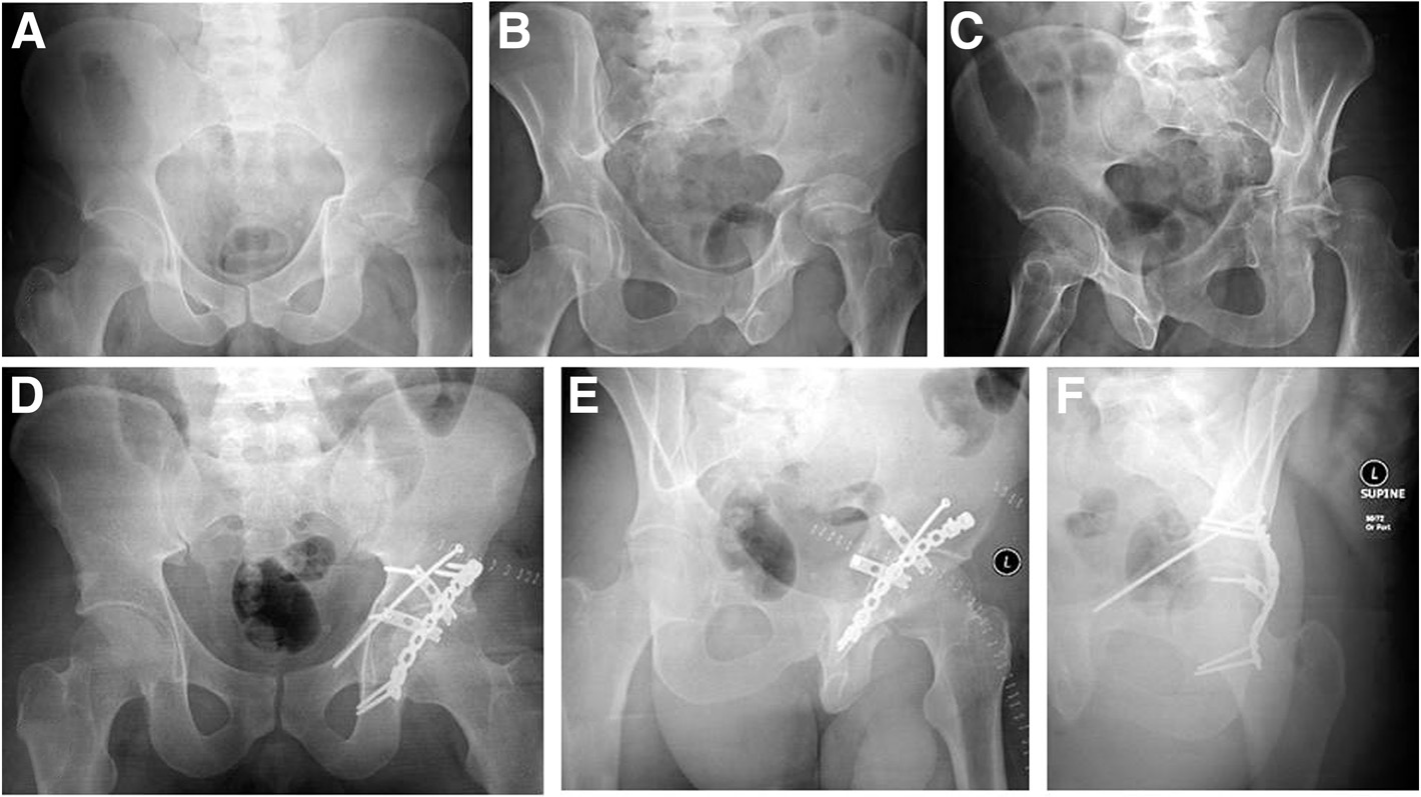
Example of an associated transverse and posterior wall fracture treated through Kocher-Langenbeck approach, using a 3.5 mm reconstruction plate, two third-tubular spring plates, and a 3.5 mm anterior column screw. (A-C) Preoperative X-rays (a.p., obturator oblique, iliac oblique). (D-F) Postoperative X-rays (a.p., obturator oblique, iliac oblique).
